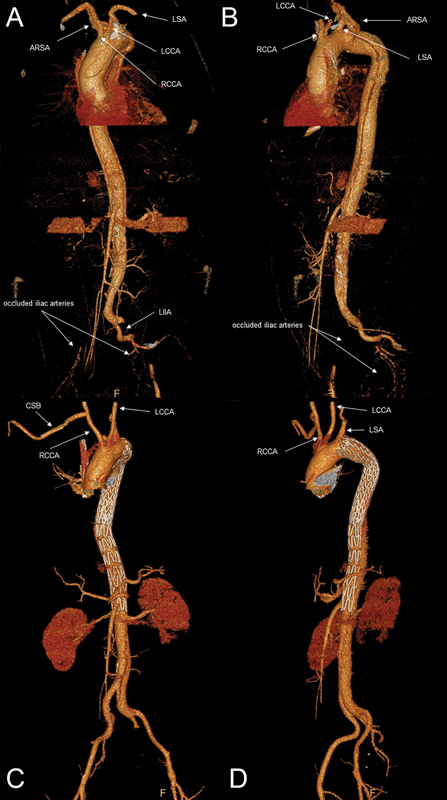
Pre- and postoperative computed tomography (CT)-angiography of a 49-year-old patient. Initial CT demonstrated a Type B aortic dissection (AD) originating just distal to the take-off of the left subclavian artery and extending into both common iliac arteries
